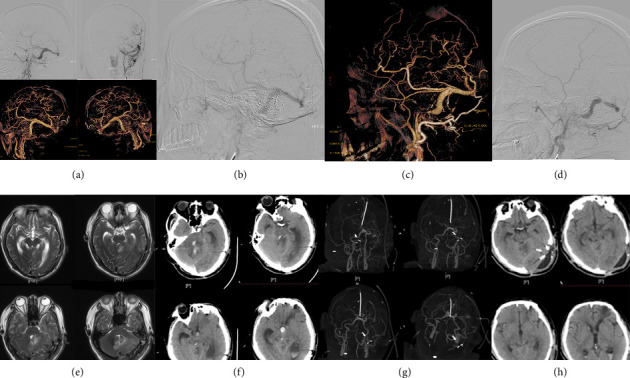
The patient, a 57-year-old male, was admitted to the hospital with a chief complaint of “headache persisting for more than three months.” (a) Preoperative cerebral angiography revealed the presence of a dural arteriovenous fistula involving the left transverse and sigmoid sinuses. (b, c) Postembolization angiographic imaging demonstrated partial obliteration of the dural arteriovenous fistula following the initial endovascular intervention. (d) Subsequent angiography after the second craniotomy, performed for definitive resection of the fistula, confirmed complete elimination of cortical retrograde venous drainage. (e) One month postsurgery, the patient developed acute severe headache. A follow-up cranial MRI revealed cerebral edema in the left cerebellar hemisphere accompanied by a small intraparenchymal hemorrhage. (f, g) The patient subsequently underwent a surgical procedure comprising right external ventricular drainage, intracranial hematoma evacuation, and cerebellar decompressive craniectomy; during this operation, the transverse sinus was ligated. (h) A cranial CT scan performed 6 months after the final surgical intervention showed no evidence of hydrocephalus, and the patient exhibited favorable neurological recovery.
